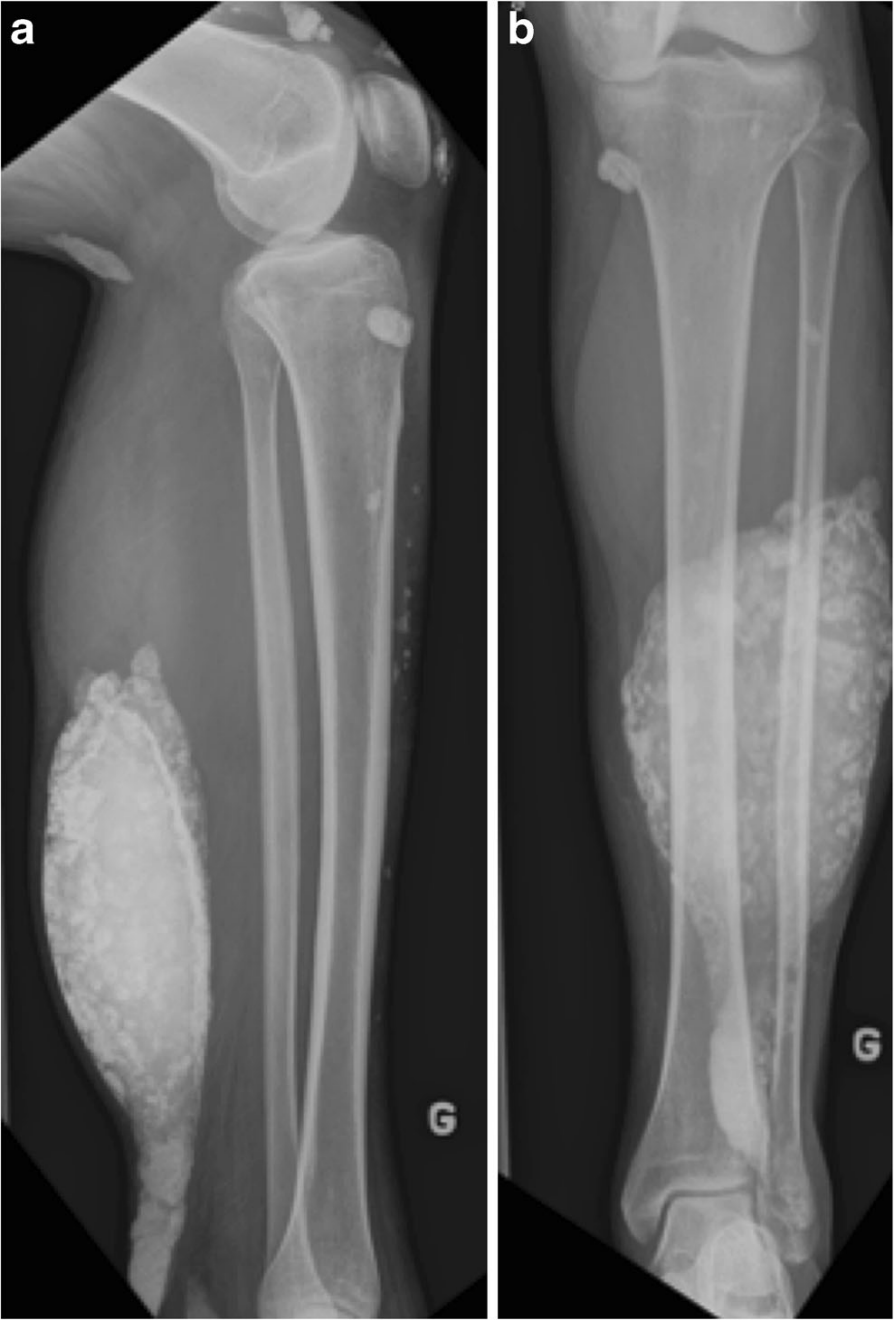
Dermatomyositis. (a) Lateral and (b) frontal radiographs of the left leg show sheet-like muscular calcifications in a case of dermatomyositis
